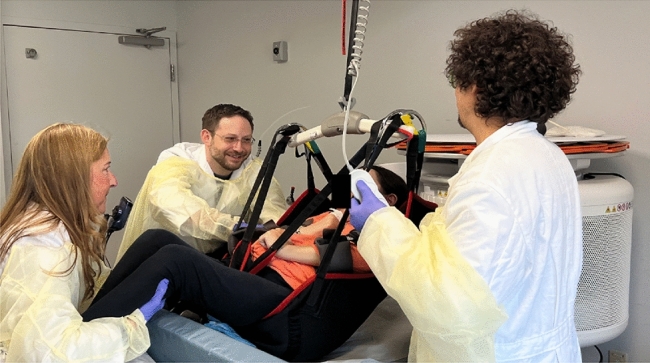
An FDA-cleared Hyperfine Swoop MRI ultra-low-field MRI (64mT), allowing for imaging of people with severe multiple sclerosis at the bedside, is shown. The Hoyer lift, which was used to safely move the individual from his wheelchair to the MRI table and facilitate proper positioning into the head coil
